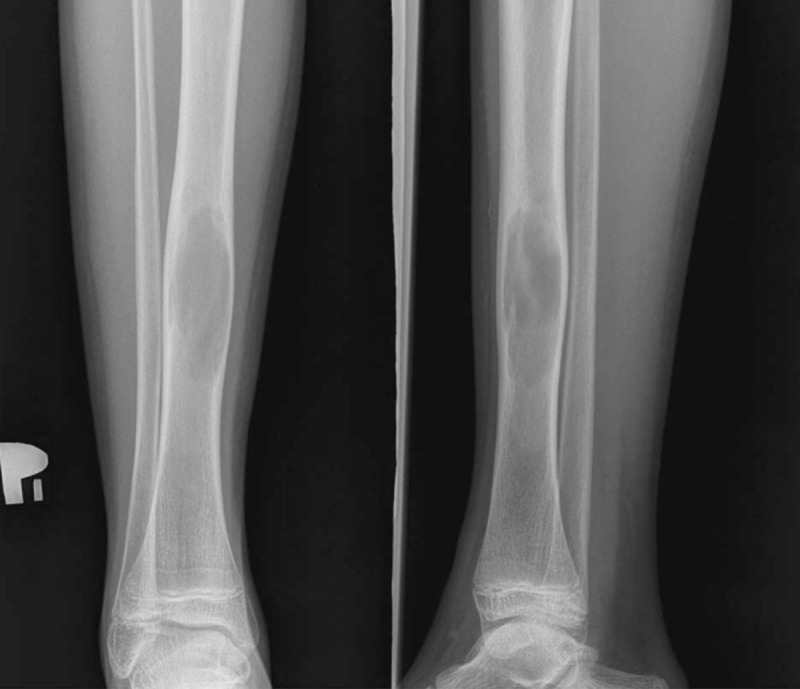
AP radiograph of the simple bone cyst of a distal/proximal tibia.
